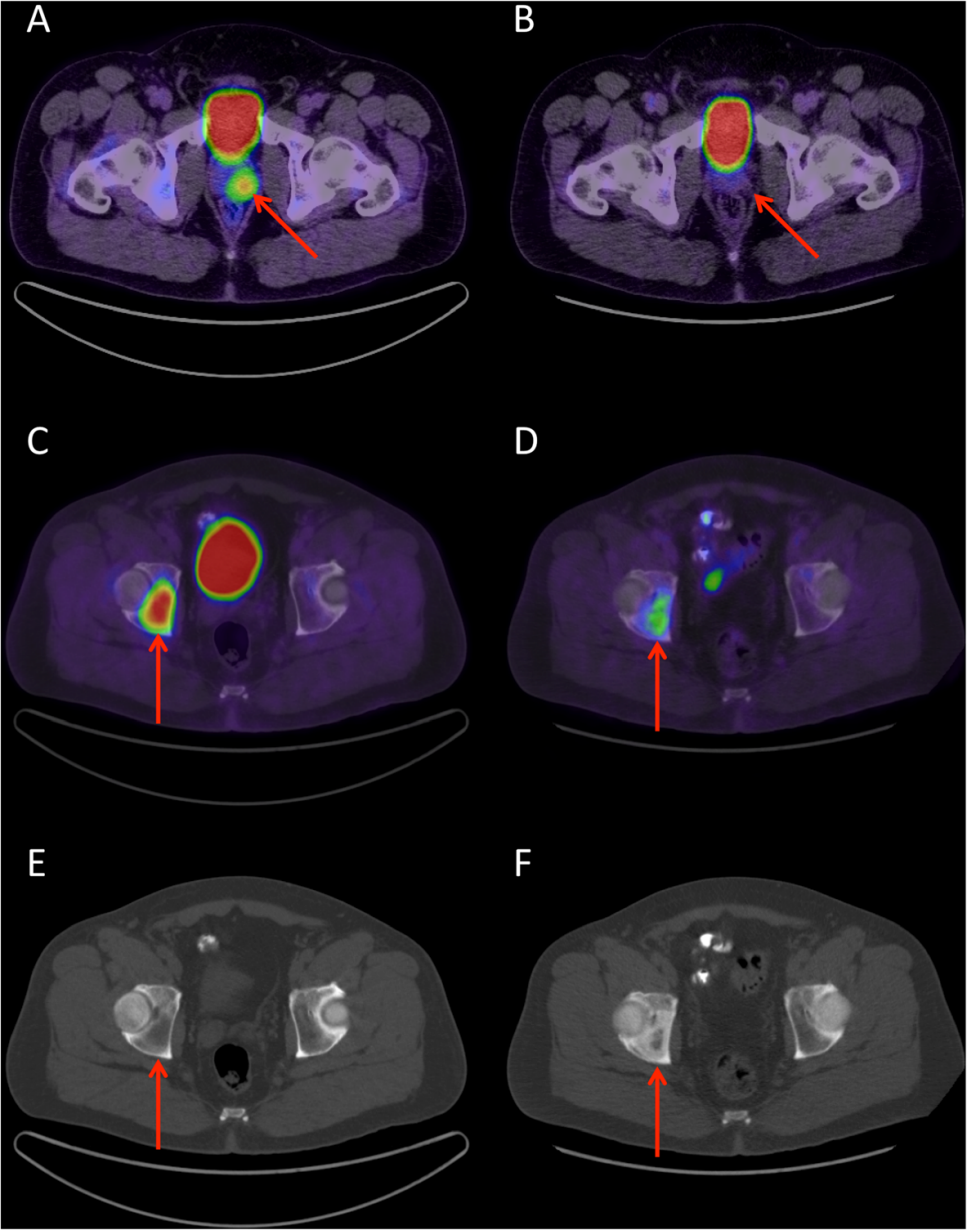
Fused FDG-PET/CT transaxial slices in a patient with a Gleason sum 9 (4 + 5) PCa at biopsy showing (A) a highly hypermetabolic prostatic focus (positive clinical report; score = 3; SUV
